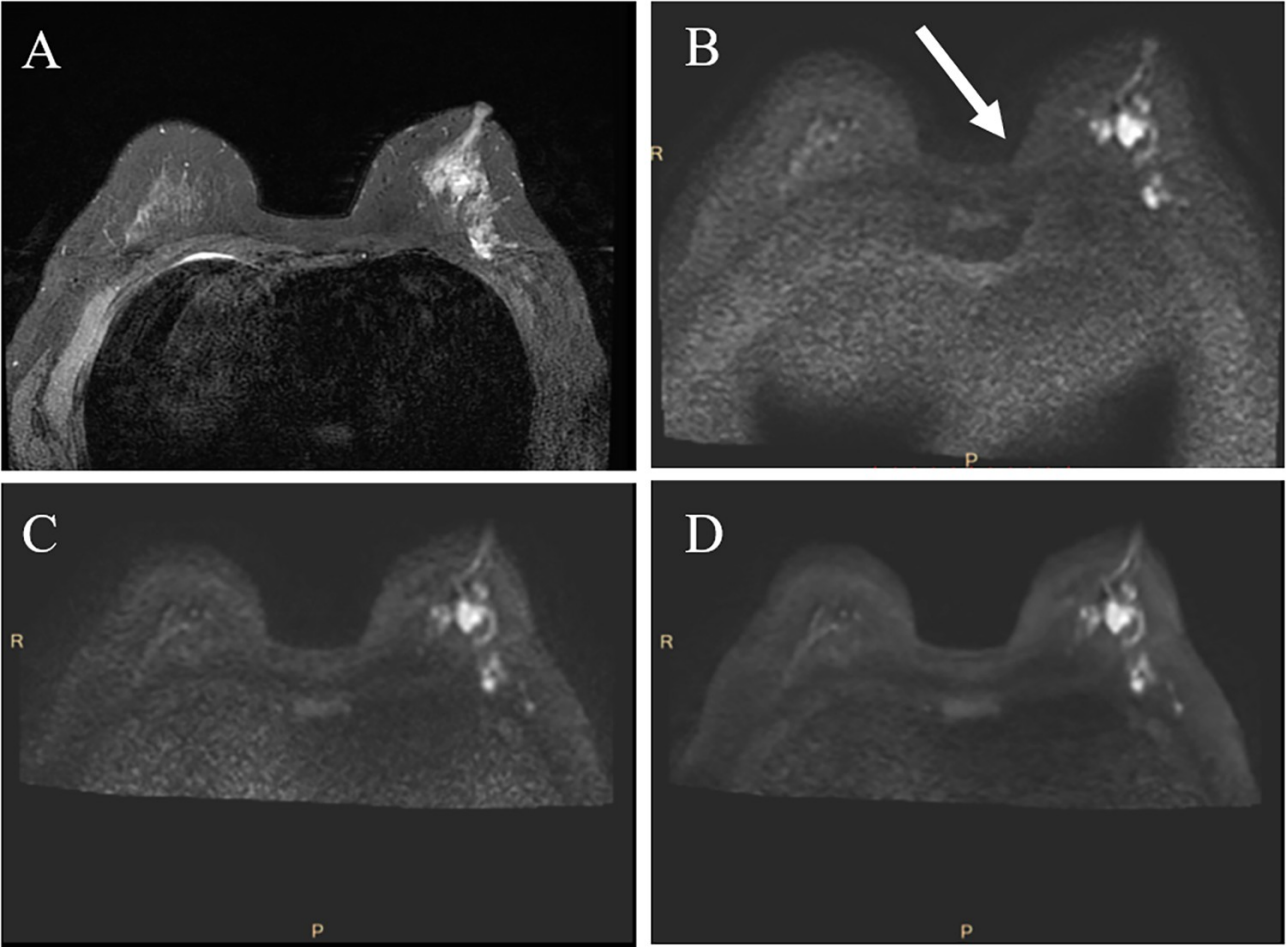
MR images of a 57-year-old patient suspected of invasive cancer in the left breast. A, T2-weighted imaging; B, conventional DWI; C, FOCUS DWI; D, FOCUS-DLR DWI. Conventional DWI exhibits noticeable ghosting, where the white arrow indicates the ghosting. However, FOCUS and FOCUS-DLR DWI show reduced ghosting. DLR, deep-learning-based reconstruction; DWI, diffusion-weighted imaging; FOCUS, field-of-view optimized and constrained undistorted single-shot.
